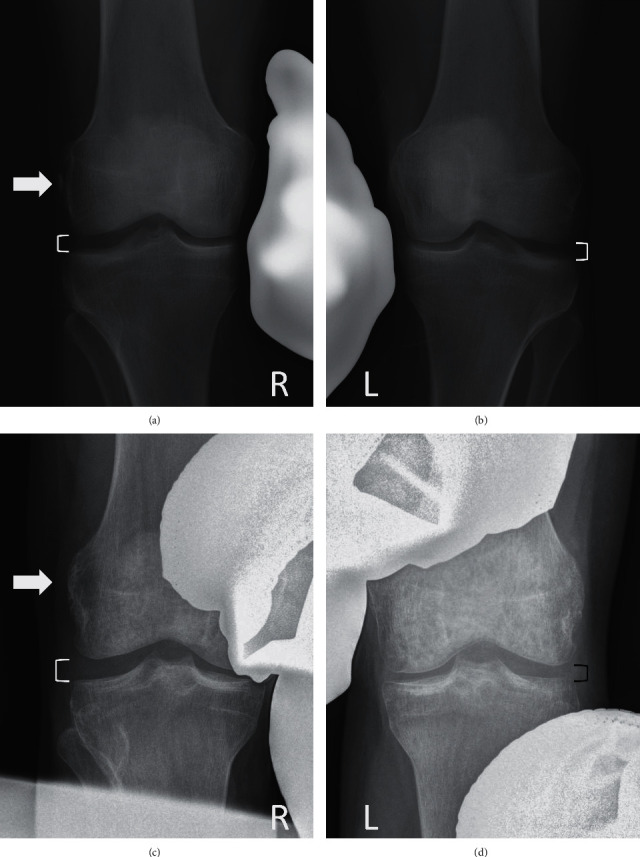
Comparative varus stress X-rays. (a and b) Patient case 1, at 14 months. Radiographic bone union of the fracture on the right knee (white arrow) as well as symmetrical side-to-side opening of the lateral compartment (white brackets). (c and d) Patient case 2, at 5 months. Radiographic bone union of the fracture on the right knee (white arrow) and 2 mm side-to-side difference between the right knee (white bracket) and the left knee (black bracket).
